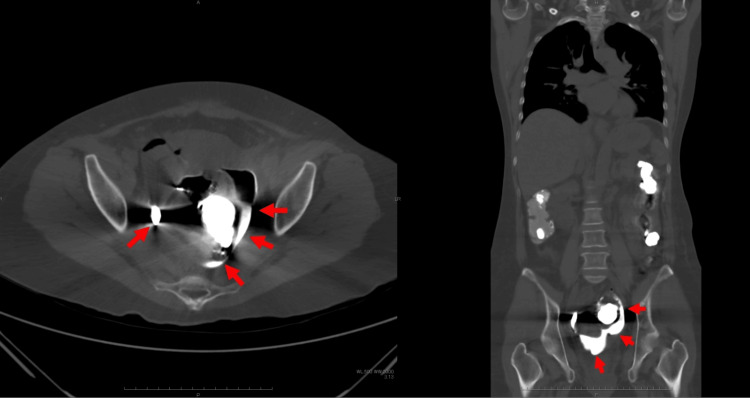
Plain abdominal CT scan A follow-up plain abdominal CT scan (axial view, left; coronal view, right) demonstrated extraluminal barium extravasation (red arrow).
